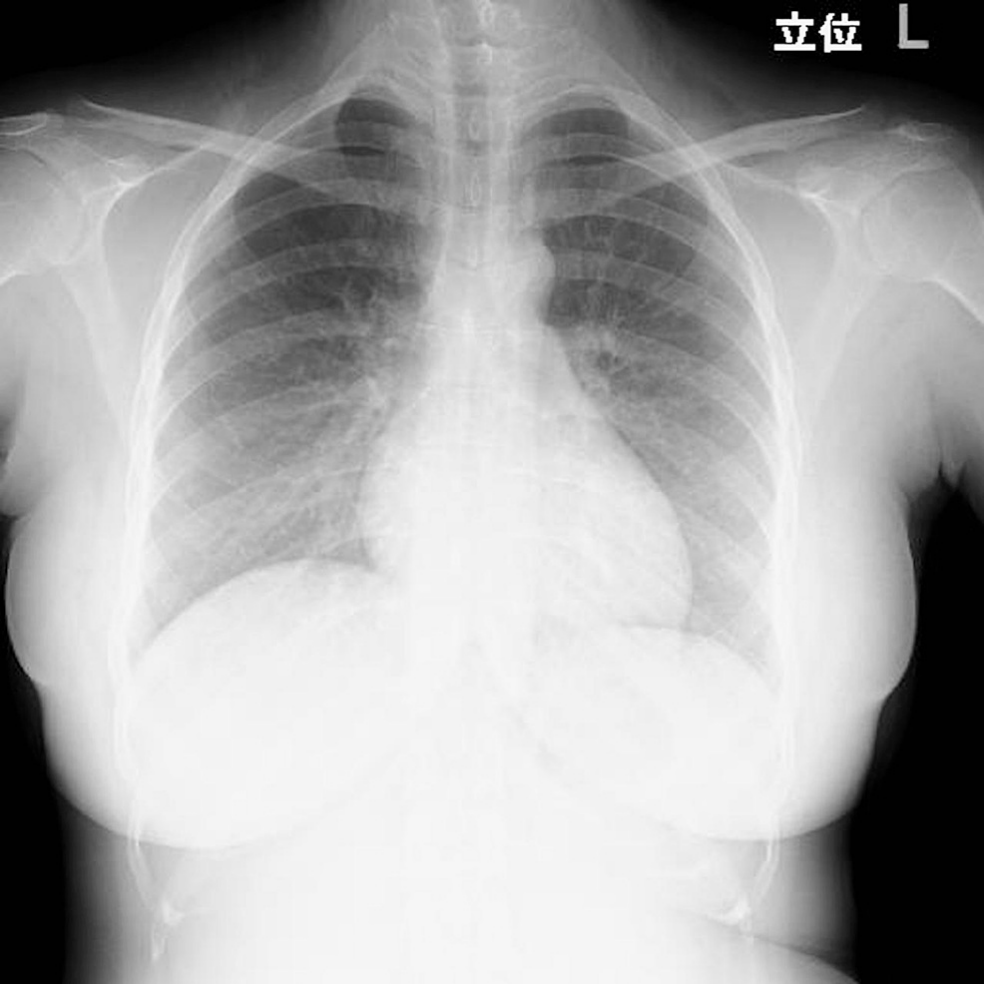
Chest X-ray image Normalized chest radiograph
